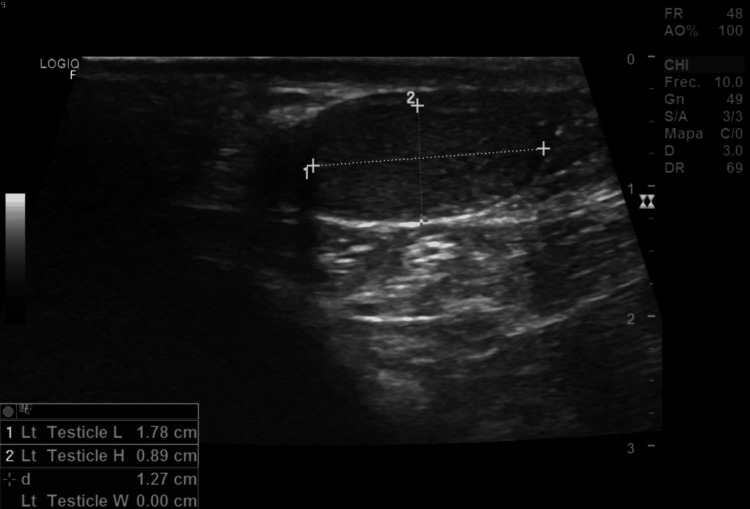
Testicular Doppler ultrasound demonstrating marked hypotrophy of the left testicle Ultrasound image showing the left testicle measuring 17 × 8 × 11 mm, consistent with significant testicular atrophy.
